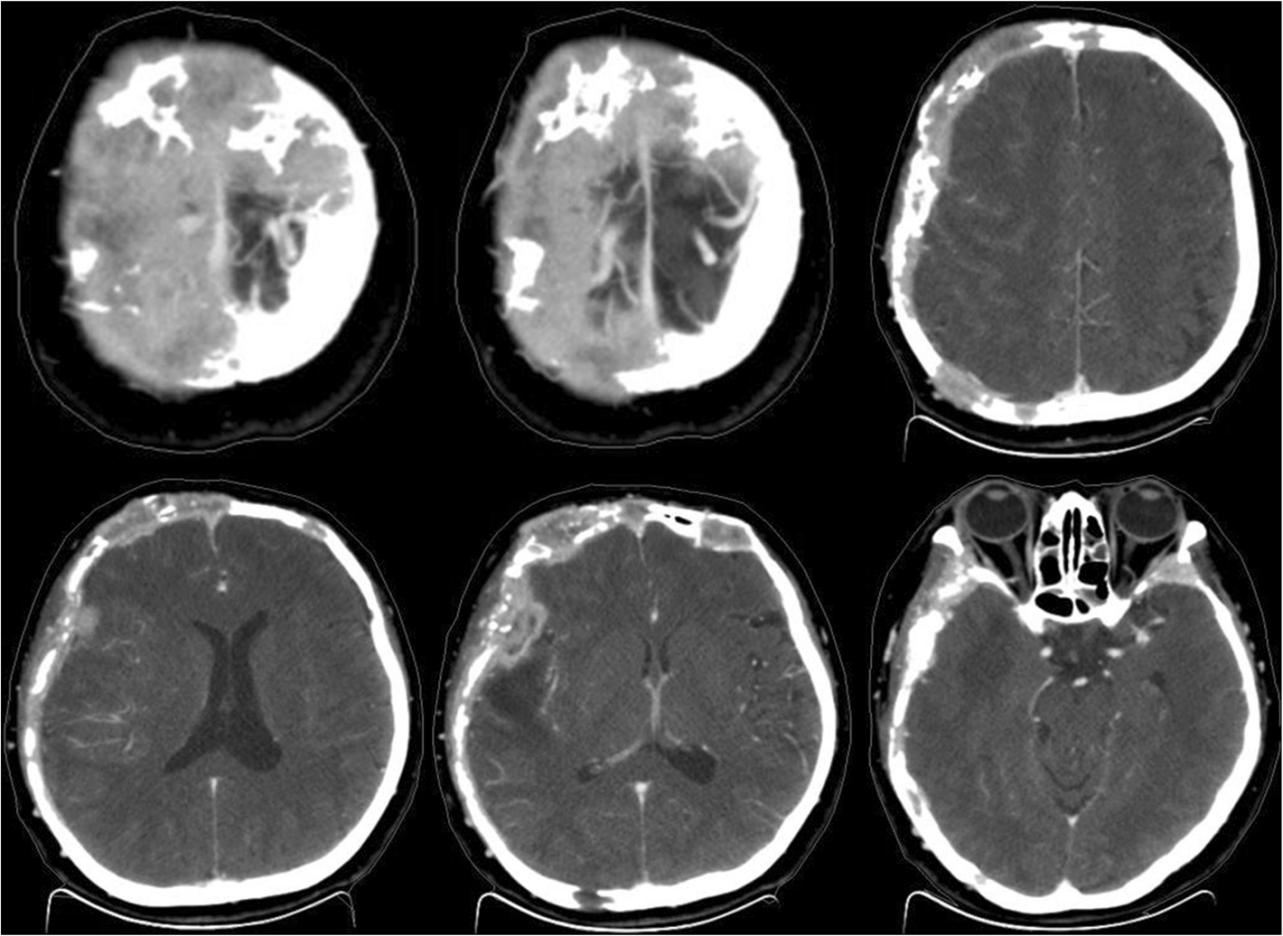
Computed tomography revealed that the right temporal lobe, and bilateral frontal lobes contained lesions. Meanwhile, the bilateral forehead, and right temporal occipital exhibited diffuse osteolytic bone destruction. The tesions had a “bean curd” type of morphology. The border of the lesion was undefined. The surrounding bone without hardening
